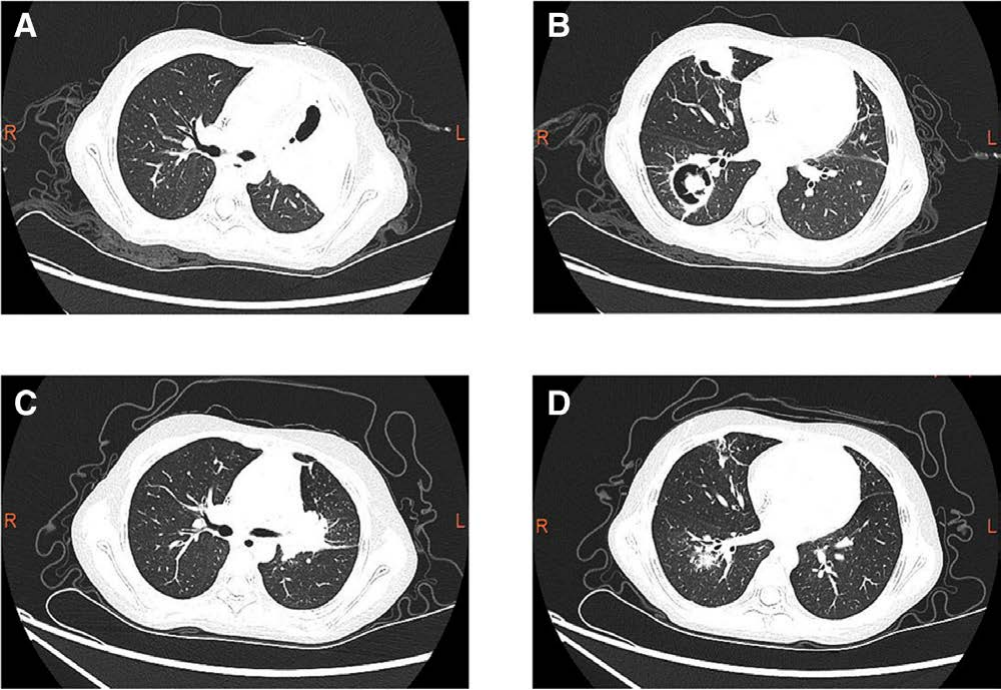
Chest CT. (A) Flaky high-density shadows with cavities in the left upper lobe. (B) Two cavitating lung nodules with “crescent sign” in the inferior lobe of right lung. (C, D) 1 month after discharge showed that the lesions were more significantly reduced. CT = computed tomography.
